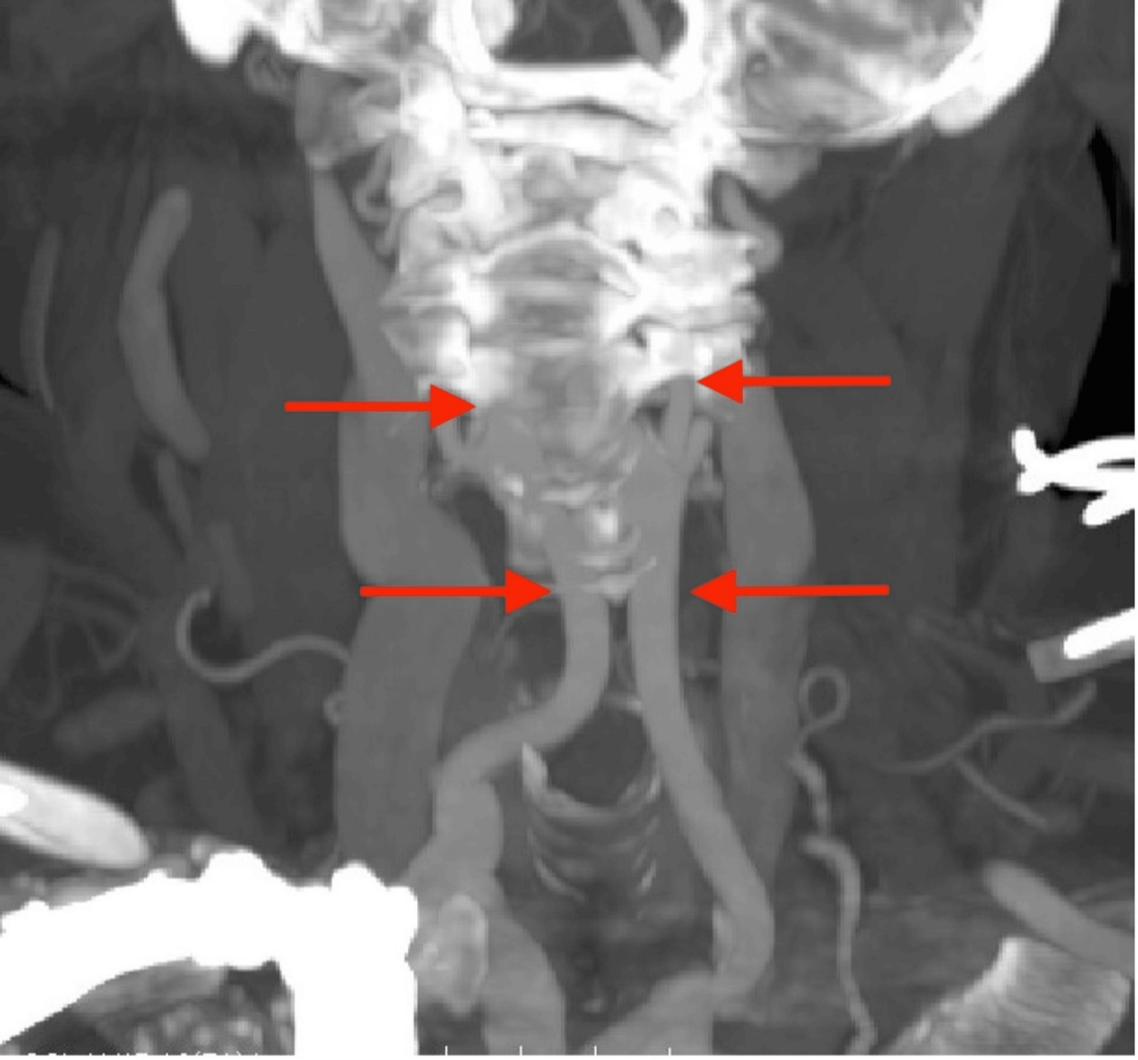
MRI of the neck demonstrates medialization of common carotids and overlying of internal carotids over the prevertebral spaces, indicated by the red arrows.
